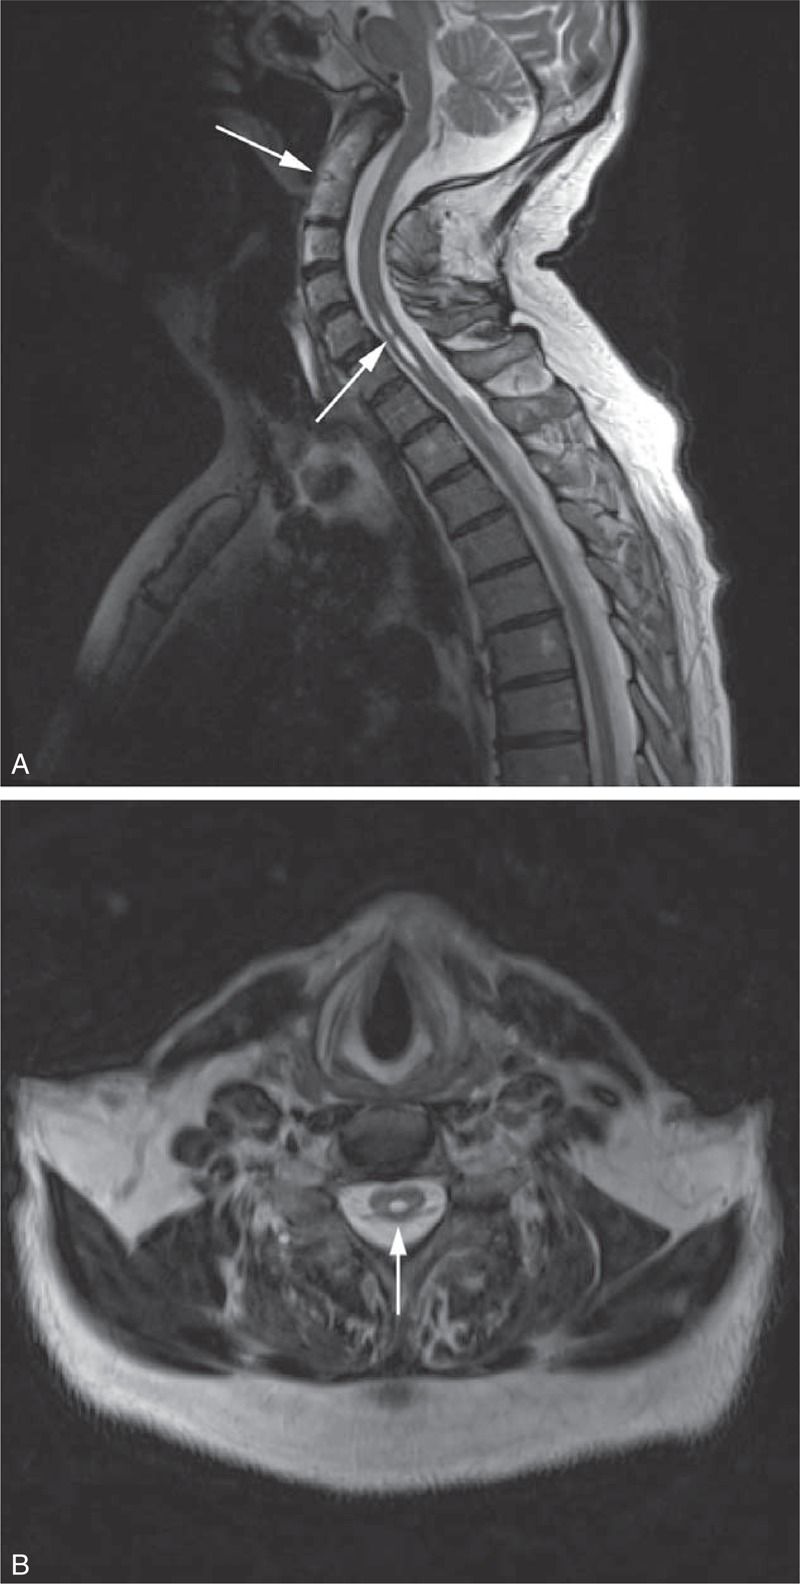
Magnetic resonance T2-weighted images. (A) Fusion of cervical vertebrae at the C2–C3 level, syringomyelia in the cervical spine (C6–C7 level). (B) Widened spinal central canal in the cervical and upper thoracic regions.
